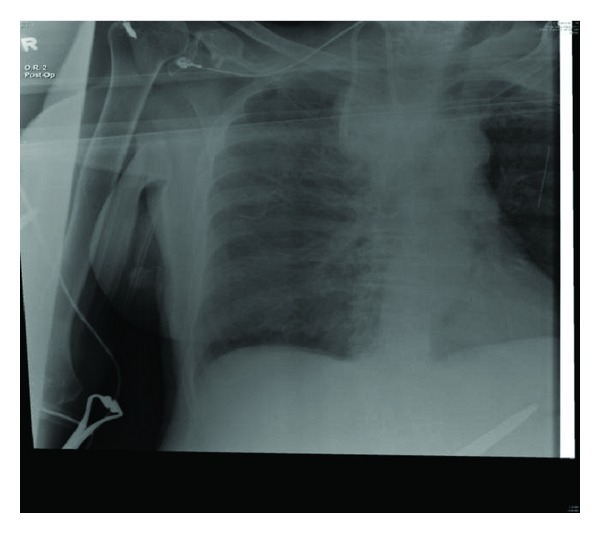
Chest X-ray showing right upper lobe whitening.
